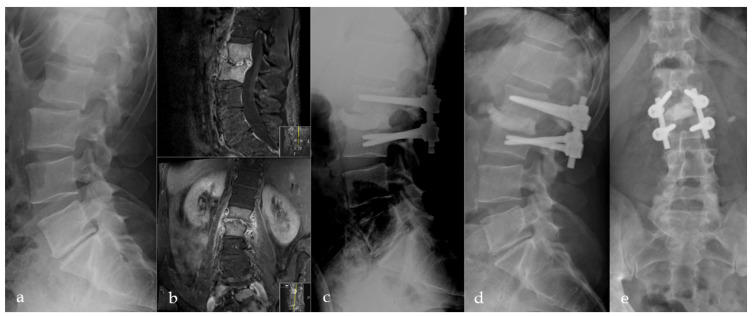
Initial X-ray shows a retrolisthesis L2/3 with mild segmental degeneration in a sixty-two-year-old male patient (a). Follow-up MRI due to persistent pain six weeks later shows pyogenic spondylodiscitis in L2/3 with progressive bony destruction (b). Directly postoperative (c) and 12 months (d,e) postoperative a.p. and lateral radiographs with anterior PMMA leakage, bone regeneration, and stable implants.
